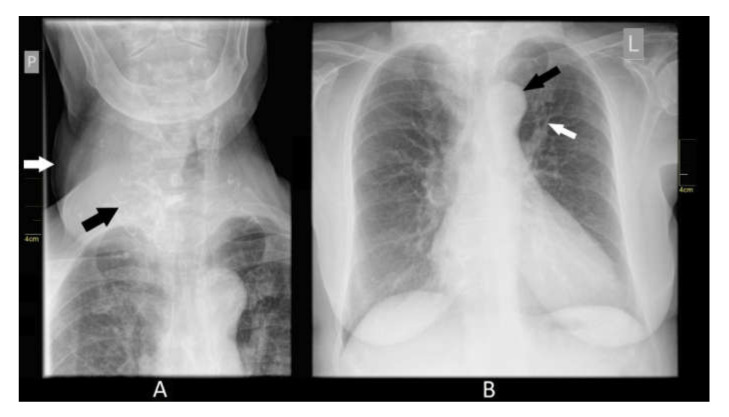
(A) X-ray of the neck, larynx, and nasopharynx. White arrow—enlarged right thyroid lobe; black arrow—calcifications; (B) Chest X-ray. White arrow—dilation of upper lobe pulmonary vessels; black arrow—thoracic aorta was elongated.
